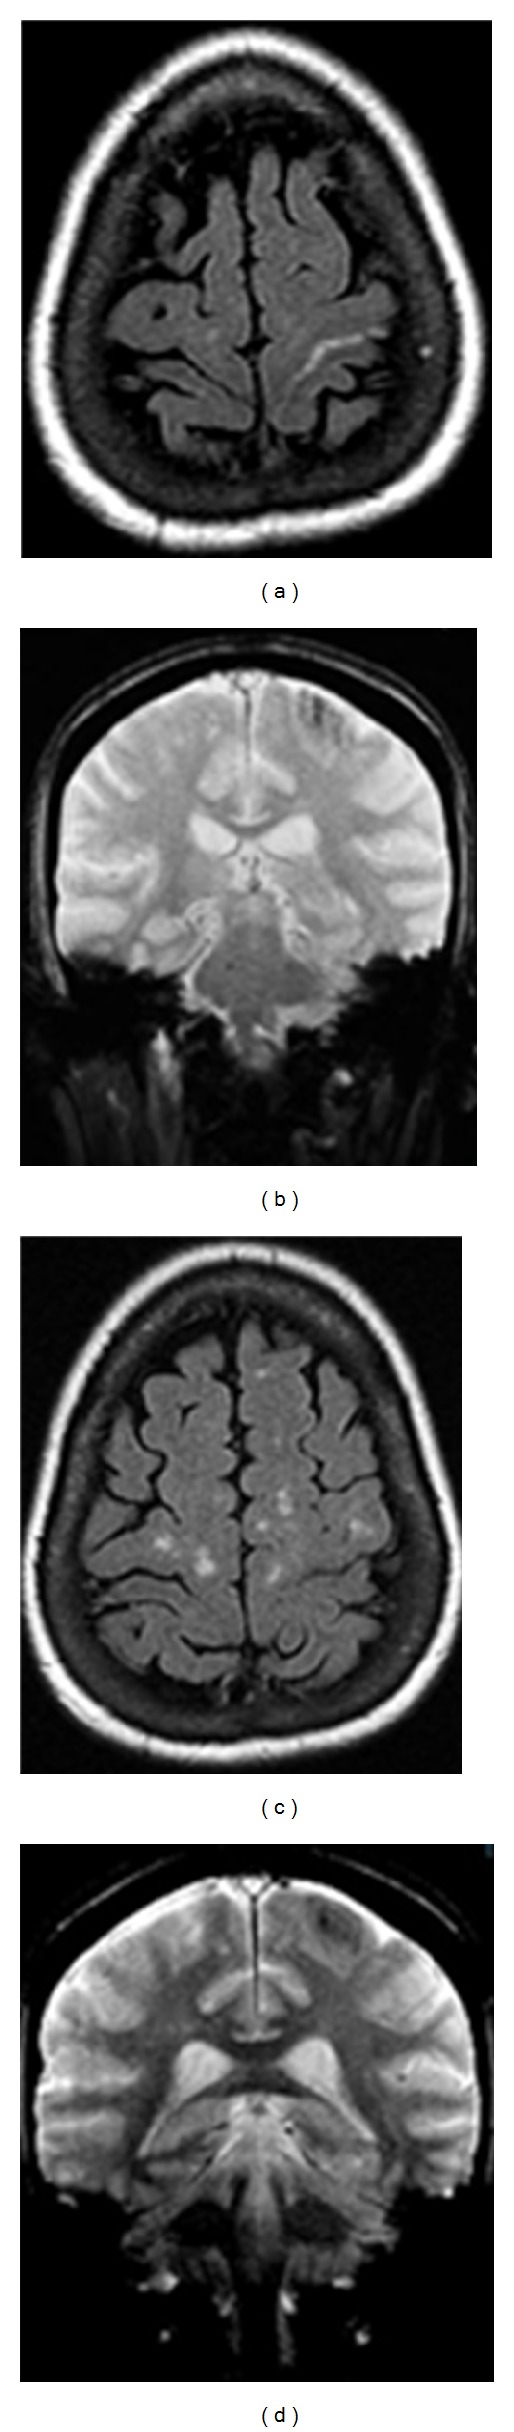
Second case of isolated central sulcus hemorrhage. MR images in a 67-year-old female with history of nasal cavity lymphoma presenting with right sided weakness. There is no history of trauma, hypertension, or bleeding diathesis. Flair MRI (image (a)) demonstrates isolated increased signal within the left central sulcus with corresponding loss of signal on gradient echo (image (b)) consistent with isolated central sulcus hemorrhage. Nonspecific white matter changes are seen on the FLAIR sequence (image (c)). MR angiogram did not demonstrate any aneurysms or vascular malformations. A probable diagnosis of cerebral amyloidosis was made based on the Boston criteria. Follow up MR images demonstrated interval resolution of the acute hemorrhage with chronic cortical and subcortical changes on the gradient echo sequences (image (d)).
